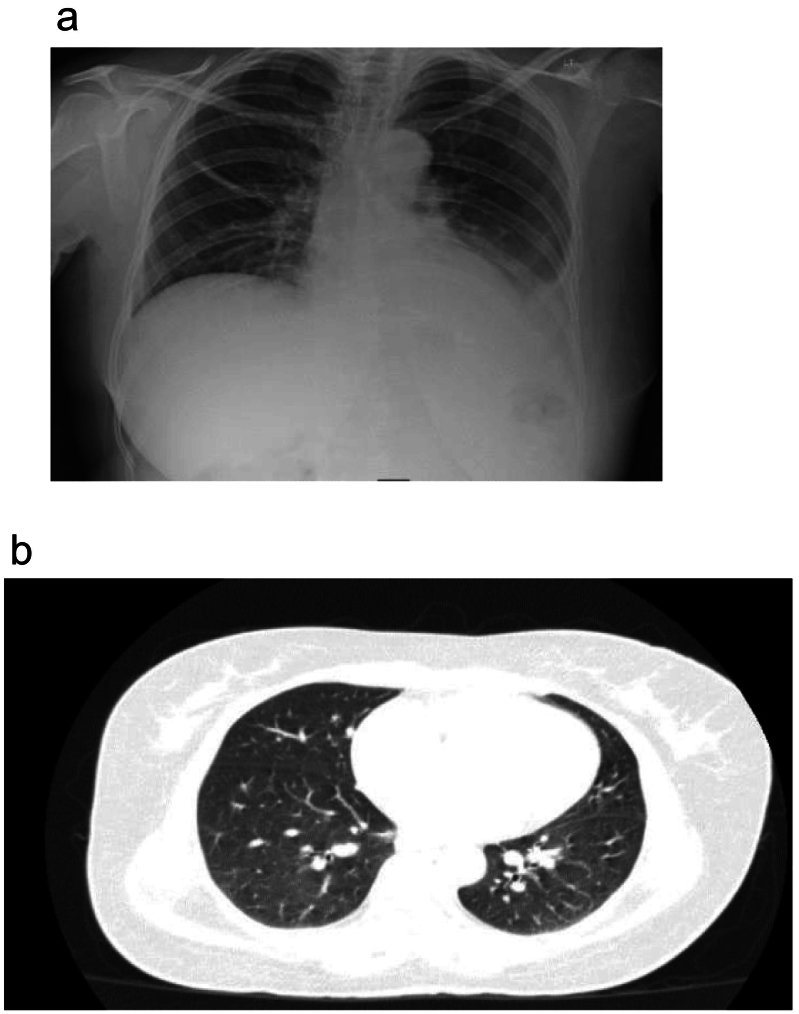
a: Chest X-ray, no features of pulmonary tuberculosis. b: Chest CT scan with no features suggestive of pulmonary tuberculosis.
